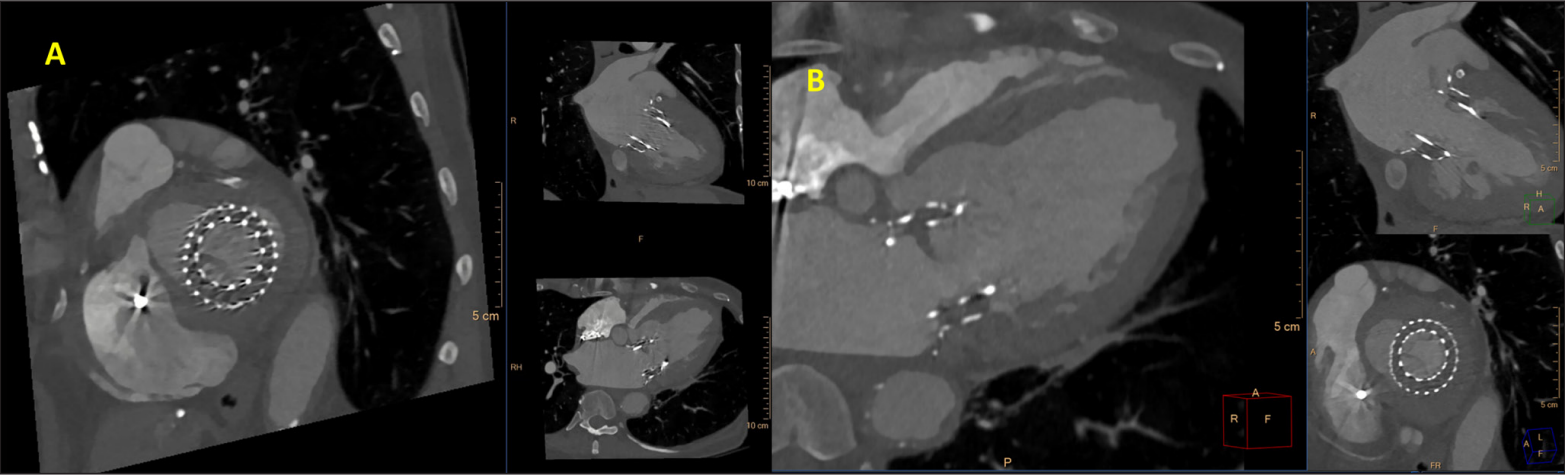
Cardiac computed tomography showing (A) video clip of the transcatheter mitral valve replacement (TMVR) with restricted leaflet motion. (B) Hypoattinuated leaflet thickening (HALT) >75% of the TMVR leaflet. See Figure 9 A videoclip at https://youtu.be/1O5I4xX9rGk.
